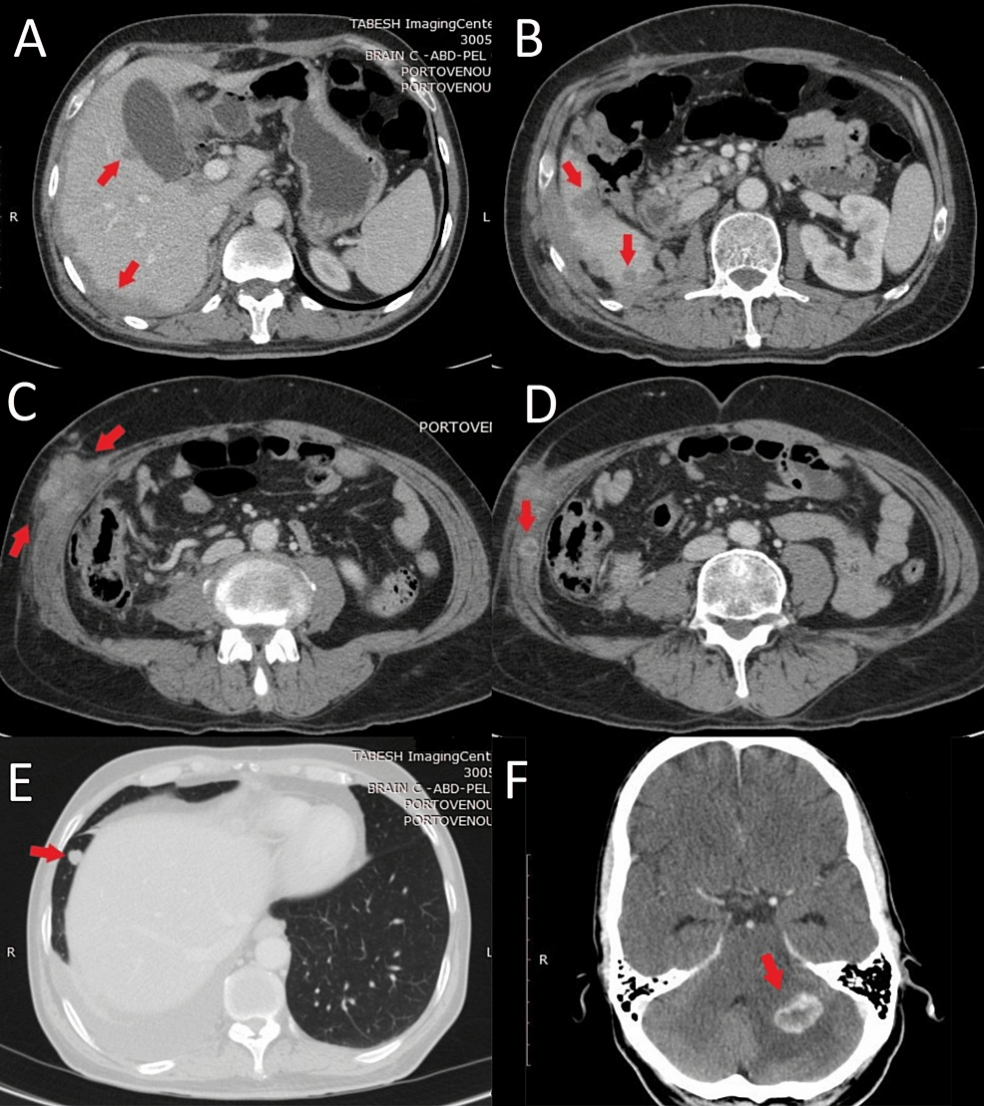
CT images for multiple metastatic lesions Multiple metastatic lesions to the (A, B) liver, (C, D) abdominal wall, (E) lungs, and (F) brain after four months of treatment with sunitinib.
